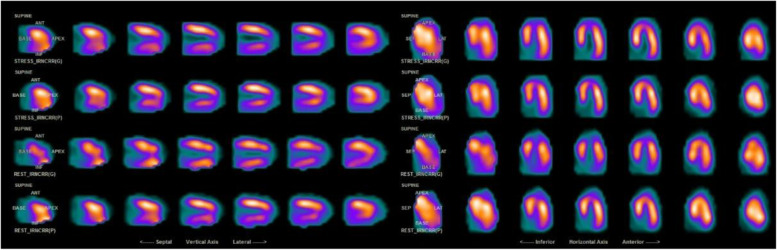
Representative image of patient’s nuclear stress test performed 2 months after admission demonstrating no radiographic evidence of ischemia
